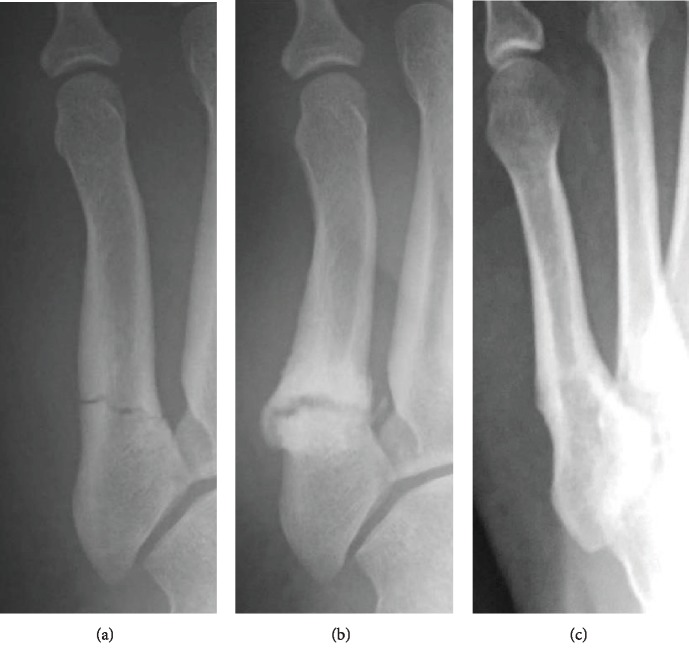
Case 1: anteroposterior radiographs of a 19-year-old sumo wrestler with a Jones fracture (a–c). At 9 months after conservative treatment, nonunion is observed on radiography (b). However, 4 years later, bone union is observed (c).
